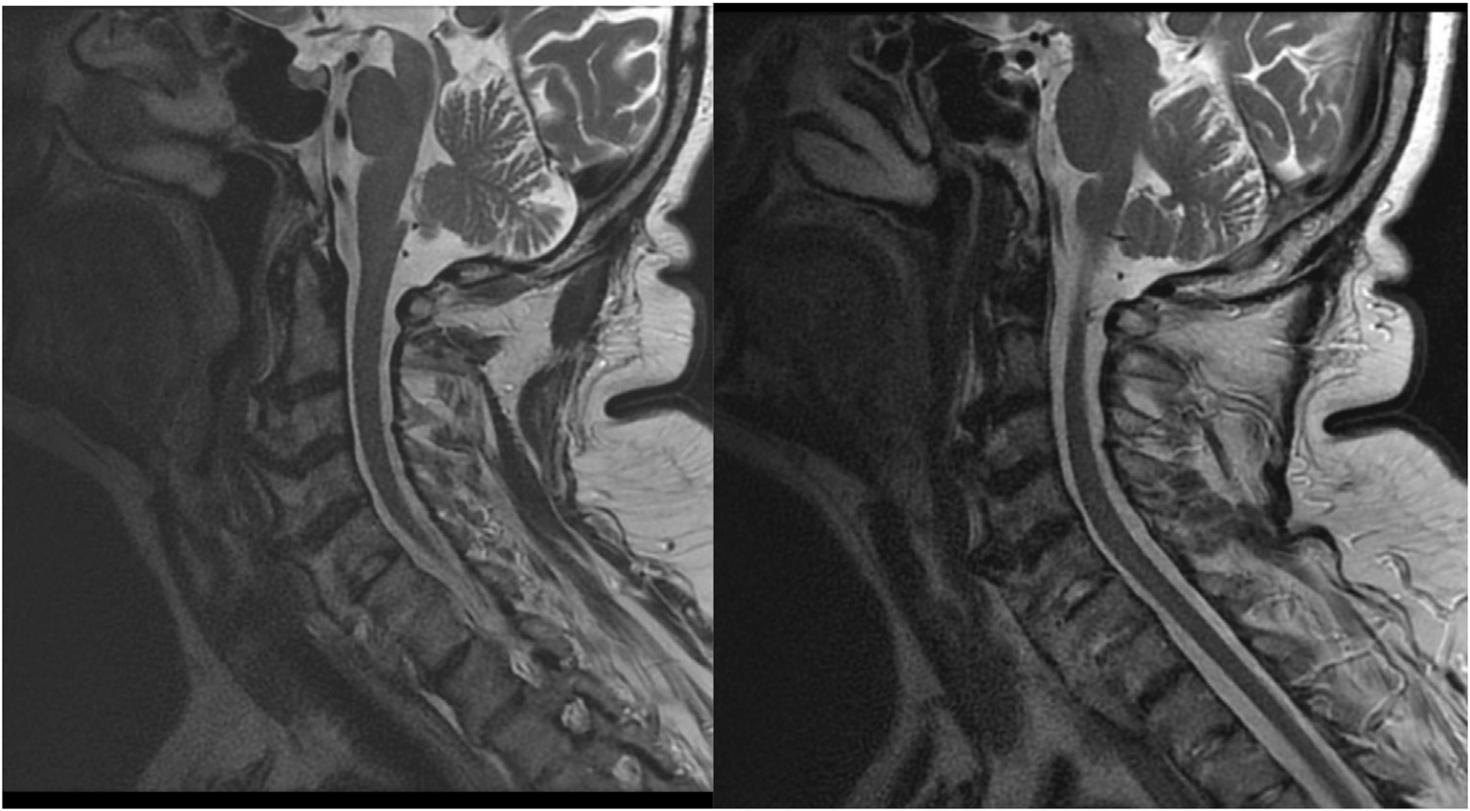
MRI scans of a 73-year-old man with diffuse idiopathic skeletal hyperostosis in cervical spine and dysphagia.
